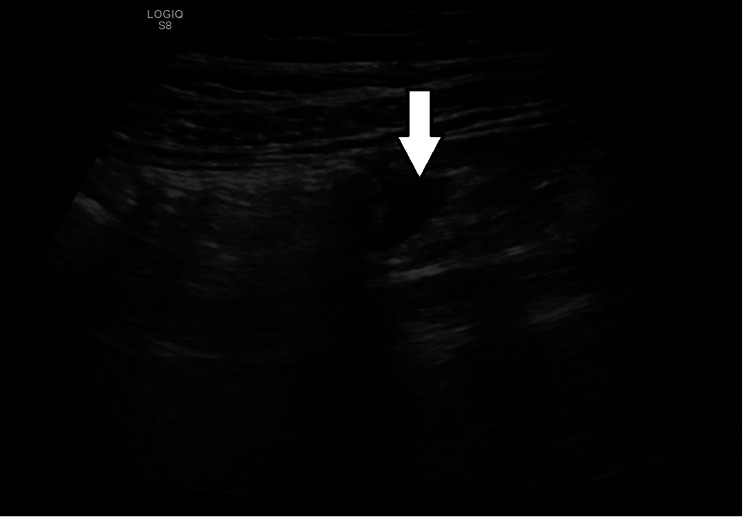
Abdominal Ultrasound Small pocket of free fluid noted in the right iliac fossa (white arrow).
